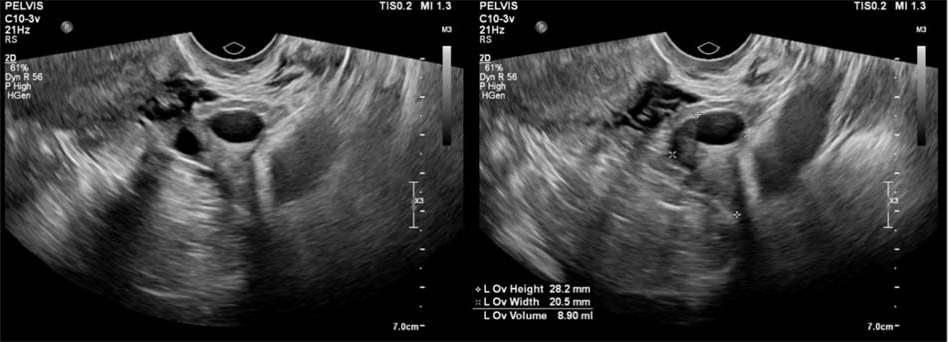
Ultrasound imaging of the right ovary, demonstrating a simple cyst.
